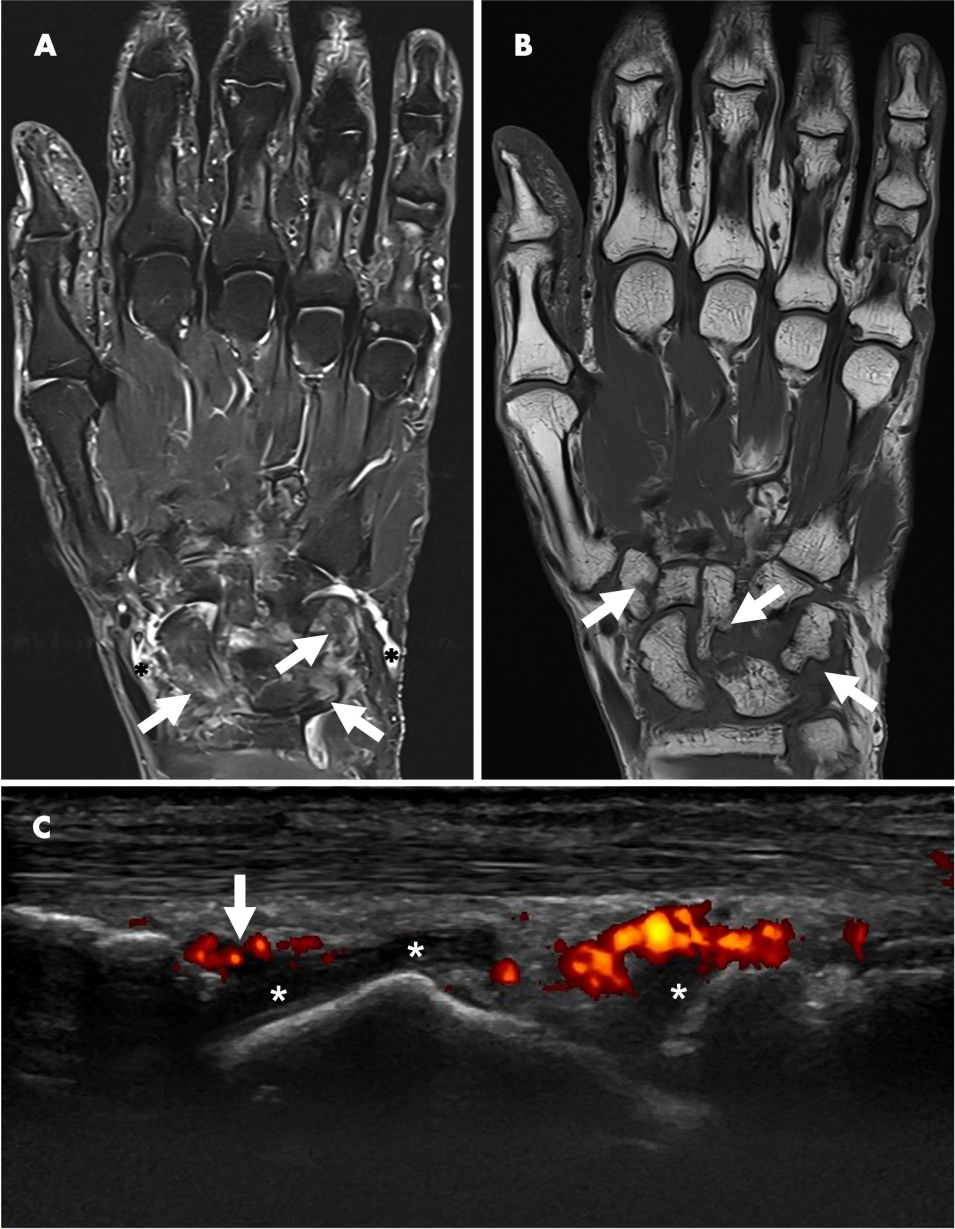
(A) Fat suppression MRI of the left wrist and MCPs showing extensive bone oedema (white arrows) and joint effusion (black asterisk). (B) T1-weighted MRI of the left wrist showing diffuse erosions of the left wrist (white arrows). (C) Longitudinal ultrasound image showing synovitis of the right wrist with grey scale (white asterisk) and power Doppler (white arrow).
